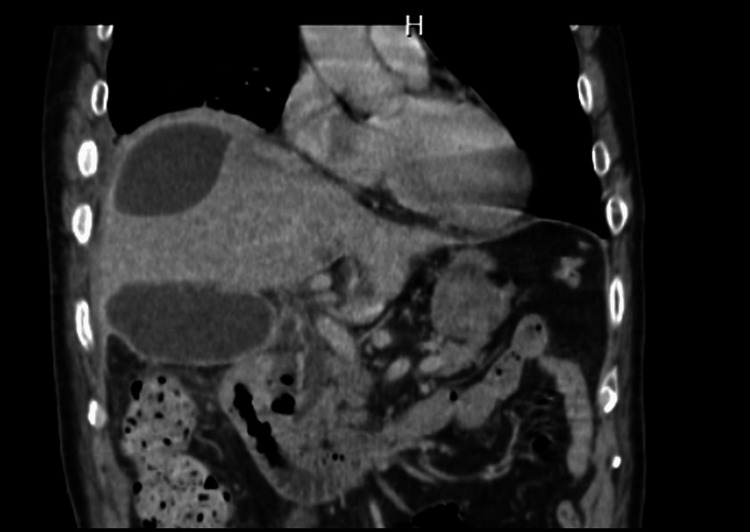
CT before initial surgery The patient had an enlarged gallbladder, wall thickening, and an abscess formation under the right diaphragm.
